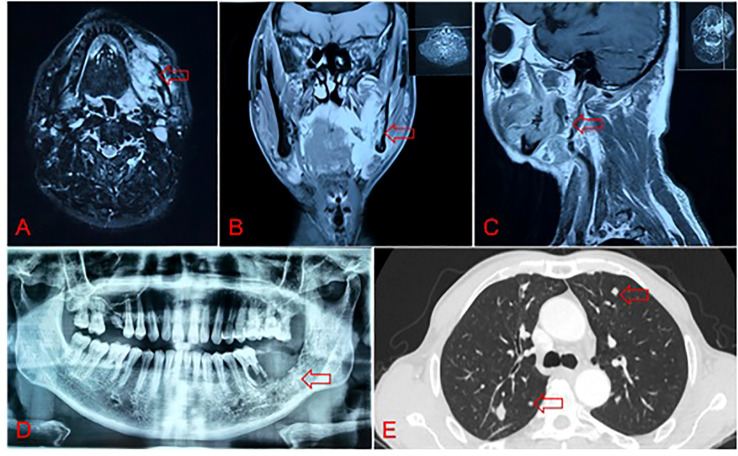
Preoperative imaging of the left mandibular lesion with pulmonary metastases. (A) Axial MRI view; (B) Coronal MRI view; (C) Sagittal MRI view; (D) Dental radiograph (periapical X-ray). (E) Chest CT scan.
